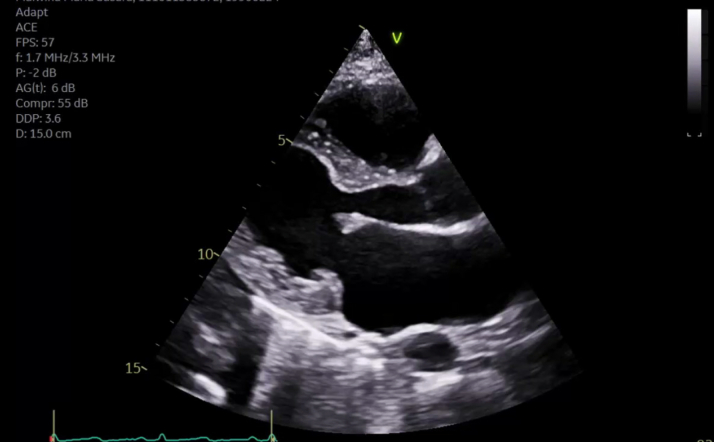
Transthoracic Echocardiography Parasternal Long Axis View Showing Resolution of Lesions
